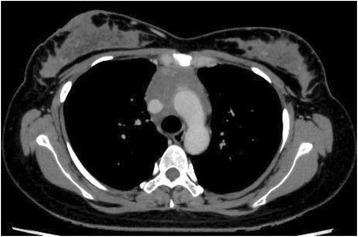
CT scan following chemotherapy showing significant regression of the mass and decreased compression of the trachea
